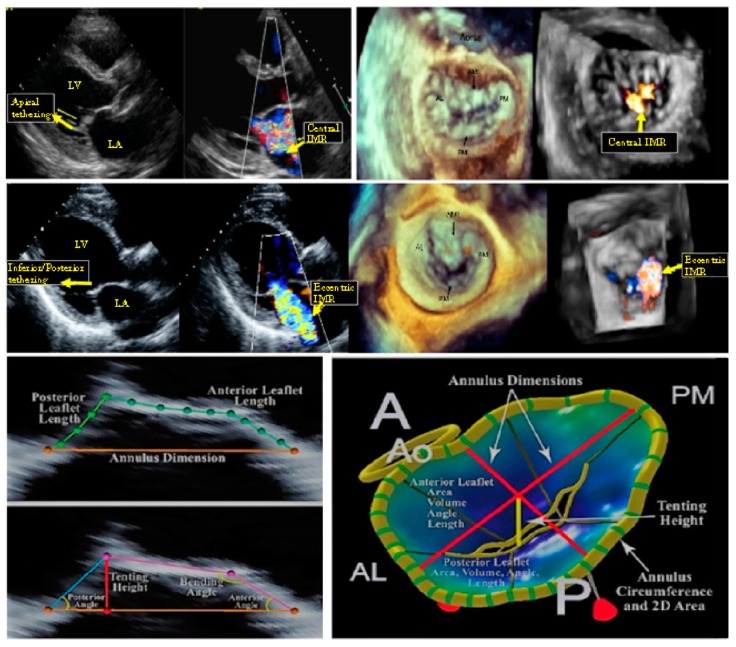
Top Panel. Symmetric pattern of mitral valve tethering determines biomechanical change in mitral valve configuration depicted on two- and three-dimensional echocardiography. (Left) Symmetric mitral valve leaflet tethering primarily in the apical direction results in a central ischemic mitral regurgitant jet. (Right) En face (surgeon’s view) of the mitral valve exemplifies a central, crescentic-shaped regurgitant orifice and MR jet. Middle Panel. Asymmetric pattern of mitral valve tethering determines biomechanical change in mitral valve configuration depicted on two- and three-dimensional echocardiography. (Left) Asymmetric mitral valve leaflet tethering in the inferior/posterior direction (yellow arrow) results in posteriorly-directed eccentric ischemic mitral regurgitation (IMR). (Right) En face (surgeons view) of the mitral valve exemplifies the resultant regurgitant orifice, which is more medially located, and the eccentric MR. Bottom panel. Two- and three-dimensional reconstruction of biomechanical change in mitral valve configuration. (Left) Representation of tenting area and tenting height. (Right) 3D TEE (transthoracic echography) reconstruction. AL = anterolateral commissure; AML = anterior mitral leaflet; IMR = ischemic mitral regurgitation; LA = left atrium; LV = left ventricle; PM = posteromedial commissure; PML = posterior mitral leaflet.
